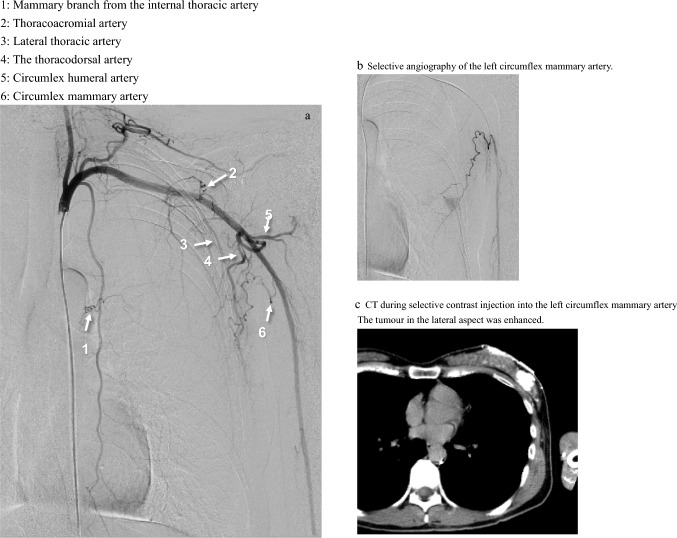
Left breast angiography and selective arterial CT. a Left subclavian digital subtraction angiography, Arrows 1: Mammary branch from the internal thoracic artery 2: Thoracoacromial artery 3: Lateral thoracic artery 4: The thoracodorsal artery 5: Circumflex humeral artery 6: Circumflex mammary artery b Selective angiography of the left circumflex mammary artery, c CT during selective contrast injection into the left circumflex mammary artery. The tumour in the lateral aspect was enhanced
